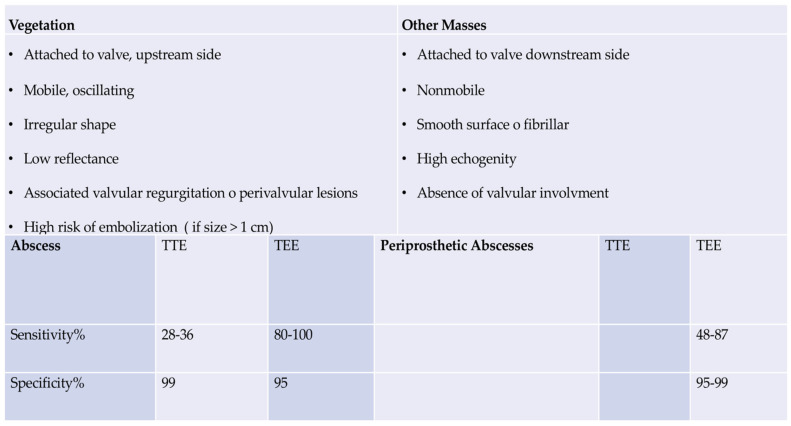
Sensitivity and specificities of echocardiography in the detection of abscesses. Refs. [27,28,31,32]. From Nappi et al. Bridging Molecular and Clinical Sciences to Achieve the Best Treatment of *Enterococcus faecalis* Endocarditis. *Microorganisms*
